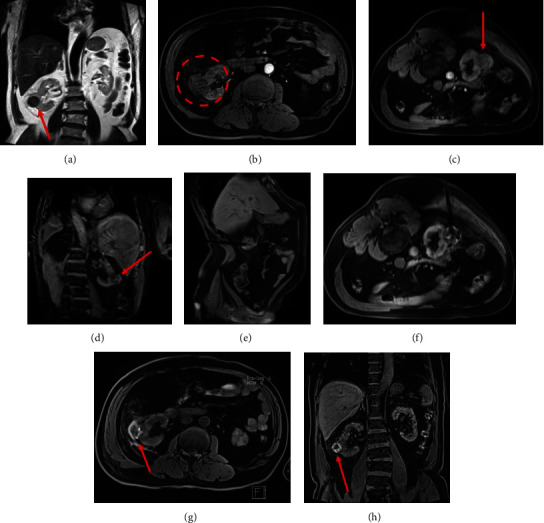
The procedure of treatment of a 54-year-old male patient with T1 renal cell carcinoma. When the patient uses T2 rapid (HASTE, 16 s) and T1 (VIBE, 16 s) sequences supine scans, it is found that the tumor is located on the right side of the body ((a) red arrow, (b) dashed circle). The lateral ablation angle cannot be implemented in the closed MRI modality, so the patient's position needs to be adjusted to find the best ablation path. Adjust the patient's body position to the oblique position ((c, d) red arrow), precisely insert the applicator into the target lesion position under the guidance of the continuous T1 (VIBE, 16 s) sequence, and ablate the lesion in the transverse position (e, f). The MWA-induced damage zone estimated as hyperintensity on T1 high signal range completely covers the tumor after during ablation ((g, h); red arrow).
